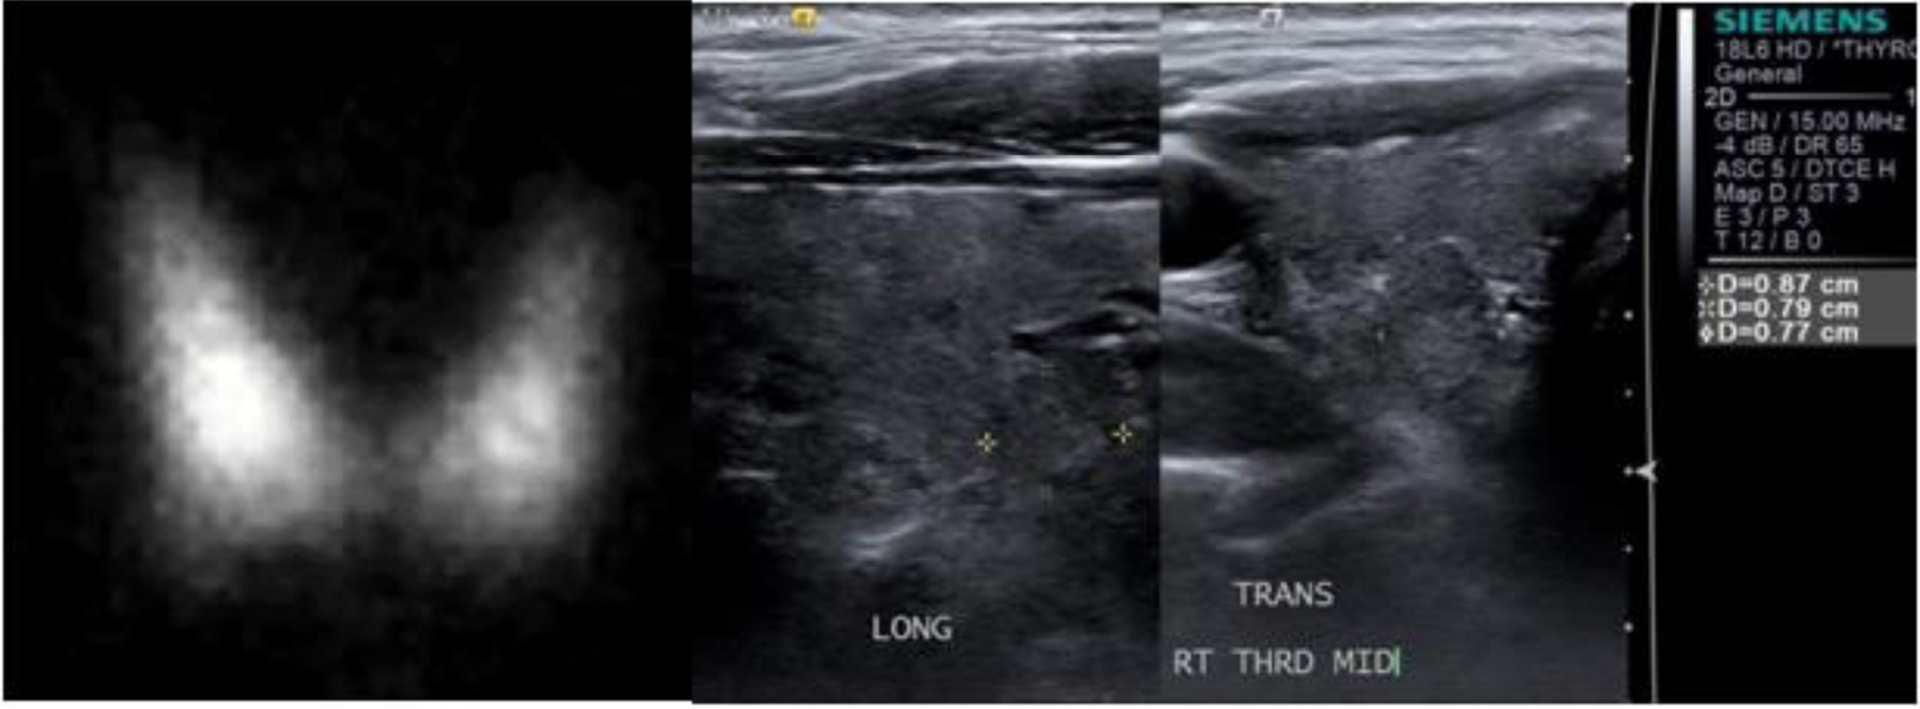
Patient B: (Left) I-123 thyroid scan on 2/26/2015 showed diffusely increased uptake bilaterally (53.9% at 24 h) and a hot thyroid nodule in the right lower lobe. (Right) Thyroid ultrasound examination on 1/13/2017. The image is the sagittal (long) and trans-axial images of the nodule itself with measurements (0.87 × 0.79 × 0.77 cm).
